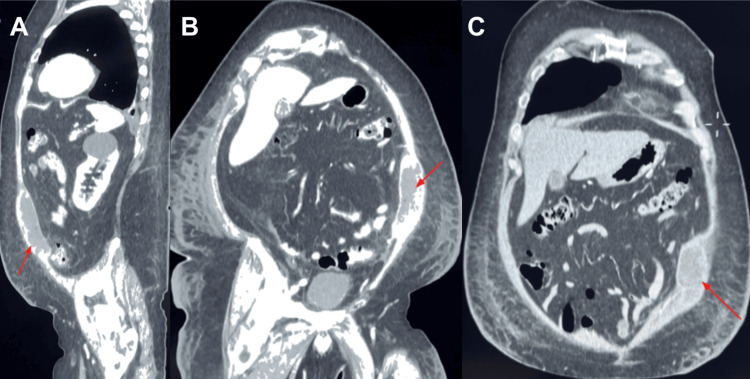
Contrast-enhanced CT images showing abdominal wall abscess. (A) Sagittal view reveals a loculated hypodense collection involving the left anterior abdominal wall (red arrow). (B-C) Axial sections demonstrate the abscess extending through the muscular layers (rectus and obliques), abutting adjacent bowel structures. The collection appears well-demarcated and heterogeneous, consistent with a mature abscess cavity (red arrows).
